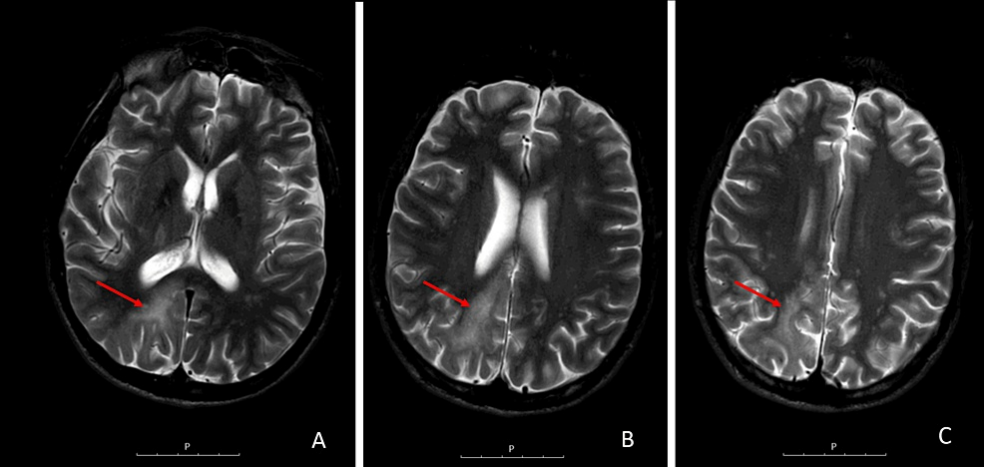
Cerebral MRI axial sections showing a right occipito-parietal lesion with hyperintense signal in T2-weighted sequence, suggesting progressive multifocal leukoencephalopathy (arrows) MRI: magnetic resonance imaging
